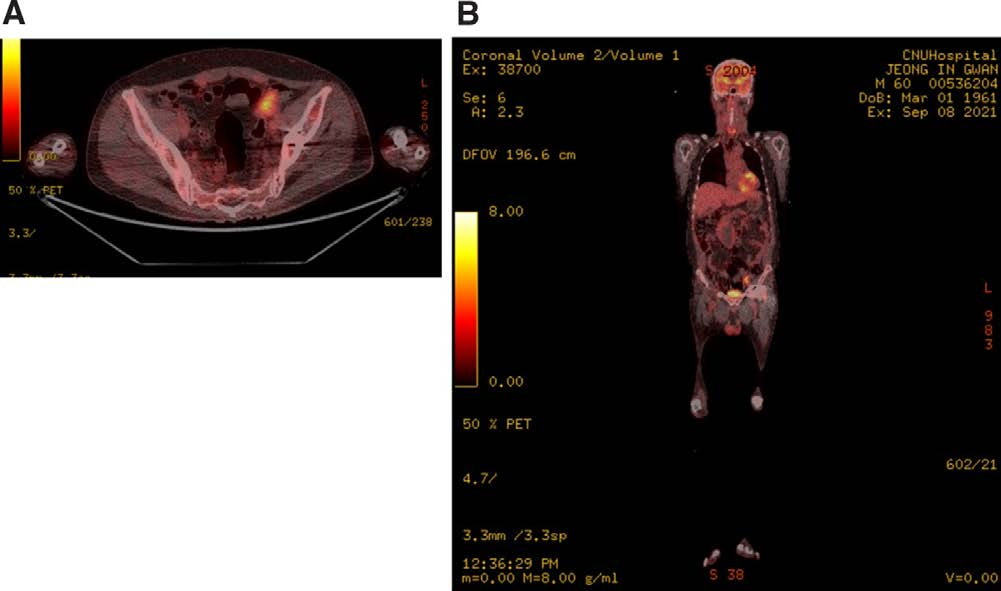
PET-CT for paraganglioma work-up. A hypermetabolic mass is observed in the proximal portion of the sigmoid colon. (5A) Axial plane. (5B) Coronal plane. PET = positron emission tomography.
